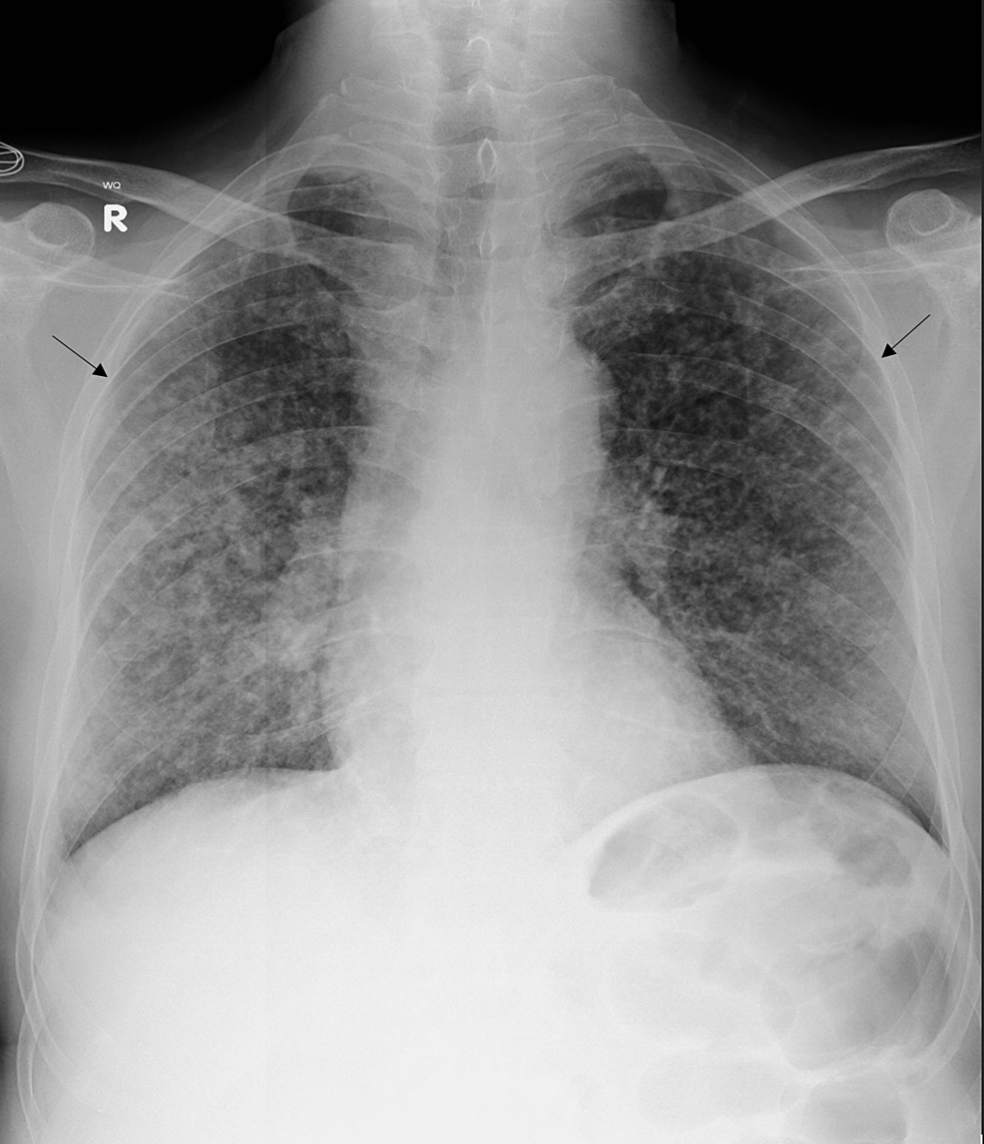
The patient's chest x-ray (PA view) showed multiple small reticulonodular nodules are seen throughout all lung lobes bilaterally. PA: Posterioanterior.
